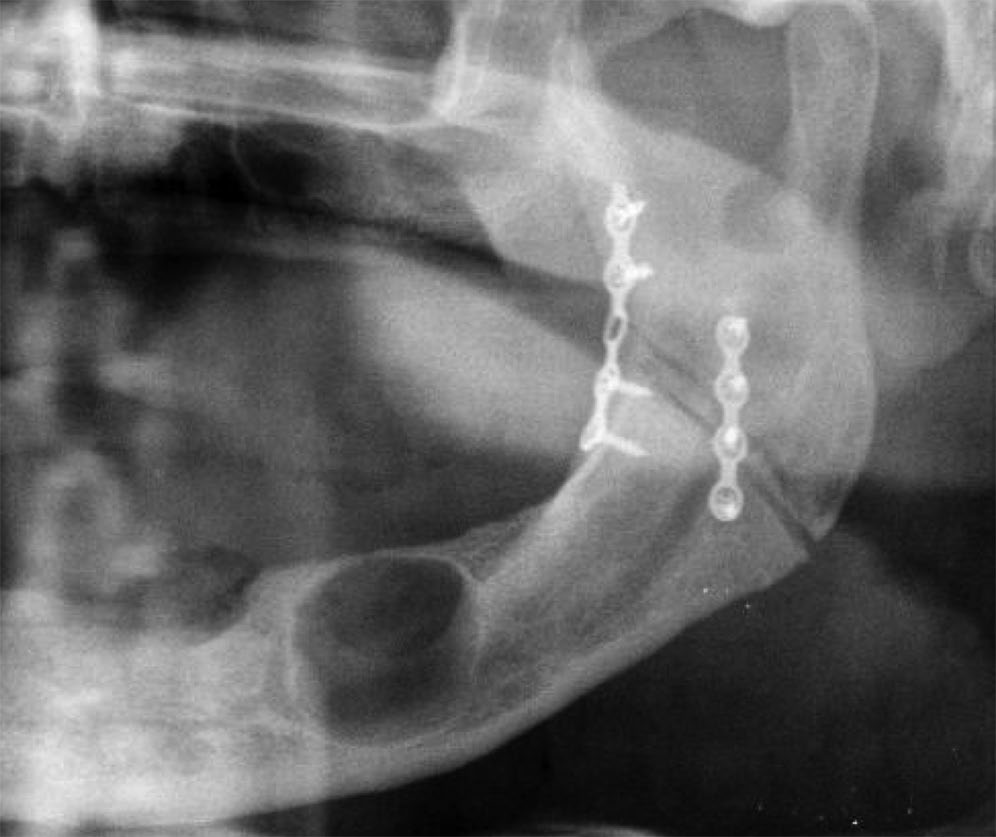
A close-up of the panoramic X-ray image of the patient received from the hospital, with the osteosynthesis in place on the left angle of the mandible.
